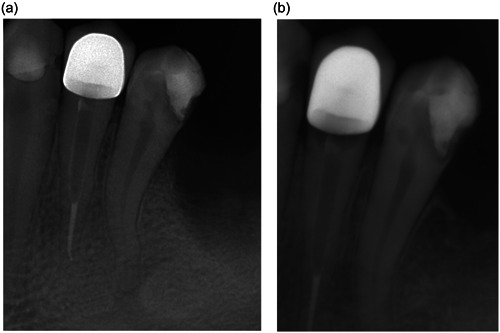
(a) Radiograph after luting. (b) Radiograph after 12 months showing a radiolucency under the crown due to post and core debonding.
